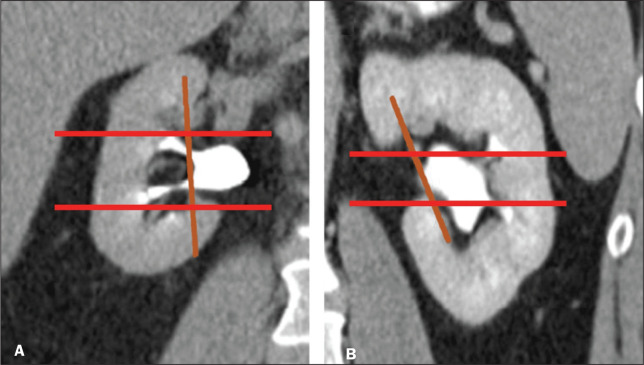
Contrast-enhanced coronal CT scans of the kidney, in the delayed phase, of two different patients, showing two types of renal pelvis: extrarenal (A) and intrarenal (B). Polar lines (red lines) drawn on each image, demonstrating the difference between the types of renal pelvis.
